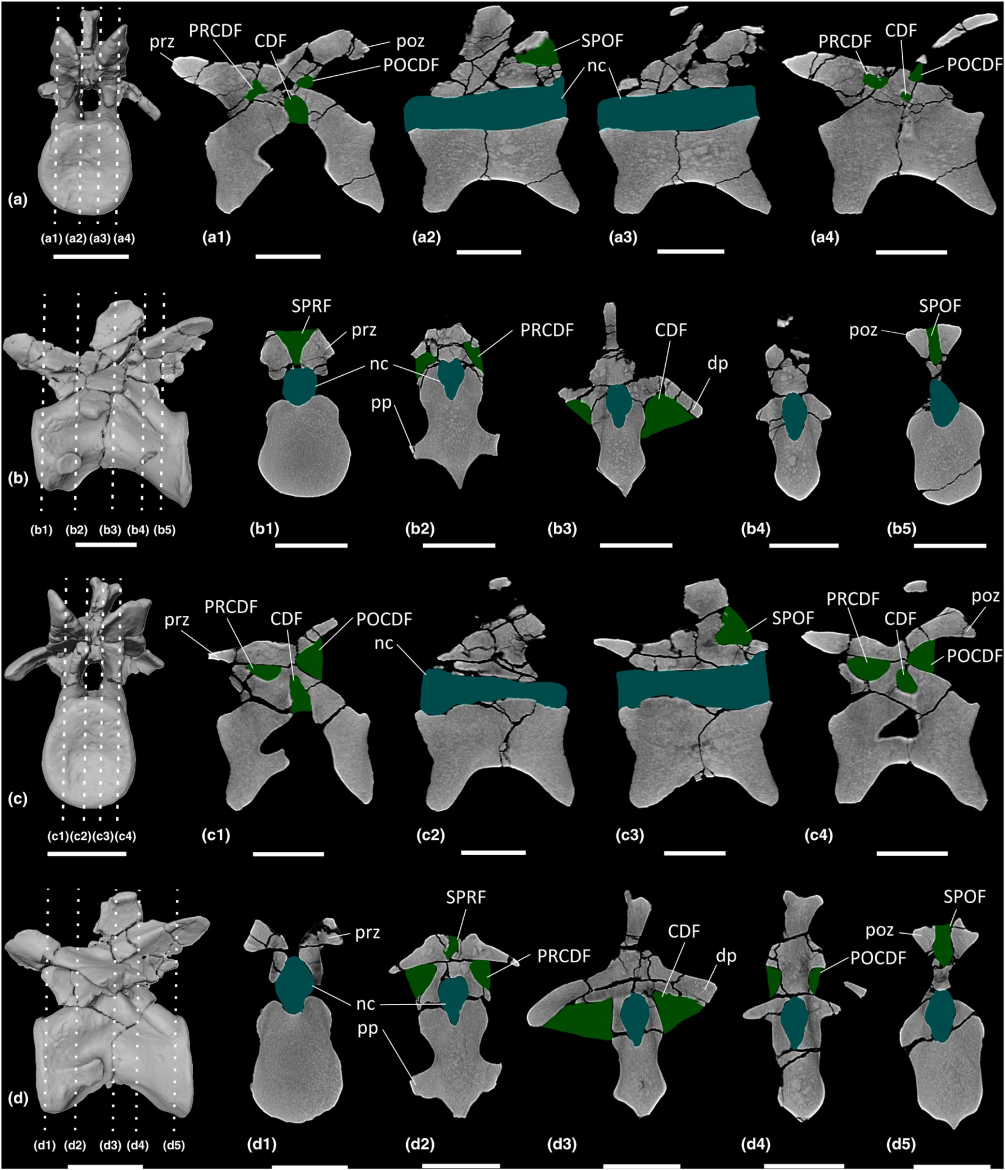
CT scan data of *Ruehleia bedheimensis* cervical vertebrae. (a, b) cervical vertebra 9 MB.R.4718.56, (c, d) cervical vertebra 10 MB.R.4718.57; (a, c) surface scan anterior view, (b, d) surface scan lateral view; (a1–a4, c1–c4) CT scan slices in parasagittal cross‐section, (b1–b5, d1–d5) CT scan slices in transverse cross‐section. CDF, centrodiapophyseal fossa; dp, diapophysis; nc, neural canal; POCDF, postzygapophyseal centrodiapophyseal fossa; poz, postzygapophysis; pp, parapophysis; PRCDF, prezygapophyseal centrodiapophyseal fossa; prz, prezygapophysis; SPOF, spinopostzygapophyseal fossa; SPRF, spinoprezygapophyseal fossa. Key: Blue highlight, neural canal; green highlight, fossa. Scale bars = 5 cm.
